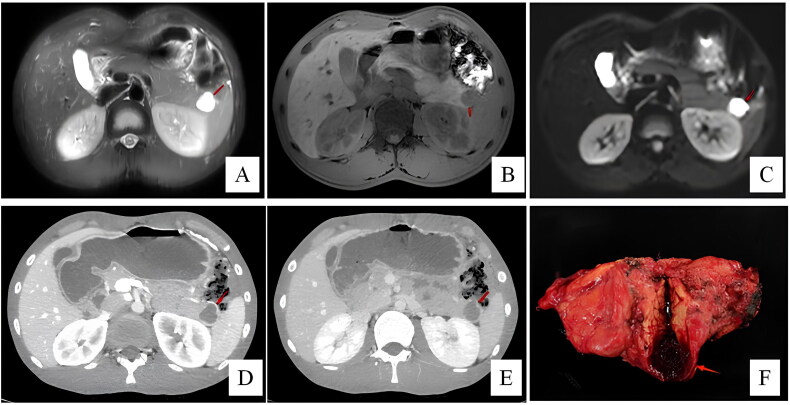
Patient 5 was diagnosed with MCN preoperative. In T2-weighted image (A), a well-demarcated cystic lesion (arrow) is seen in the pancreatic tail. The lesion (arrow) is hypointense in the T1-weighted image (B). The lesion (arrow) presents high signal intensity in DWI image (C). In arterial (D) and portal-venous phase (E), the lesion is hypointense (arrow). Macroscopic (F) image of epidermoid cyst in intrapancreatic accessory spleen (ECIPAS).
